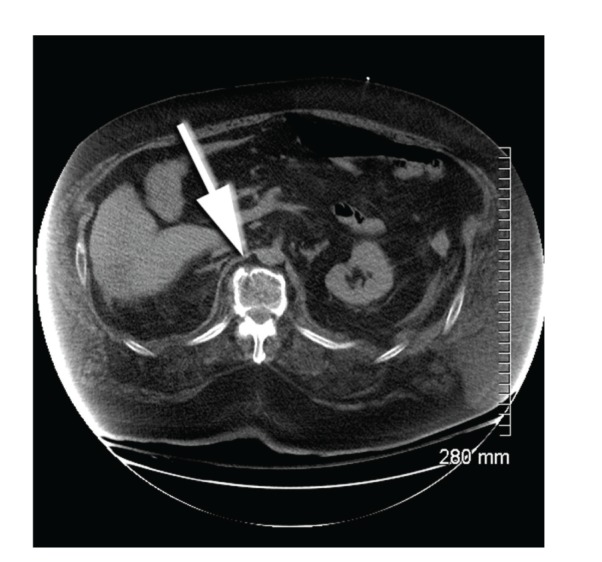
CT of case report 2 showing location of thoracic fracture.
